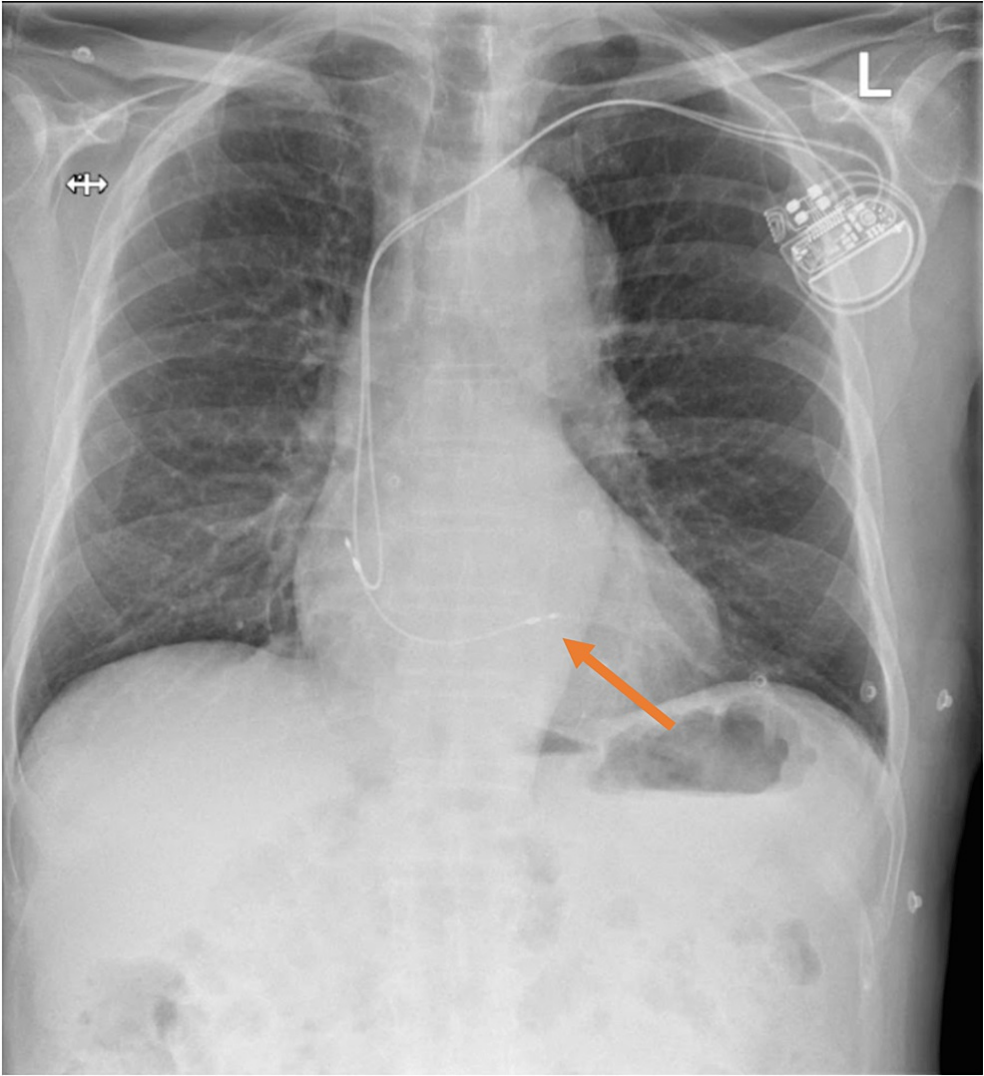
Chest radiograph showing the position of the RV pacing lead post PPM insertion. PPM: permanent pacemaker; RV: right ventricular.
